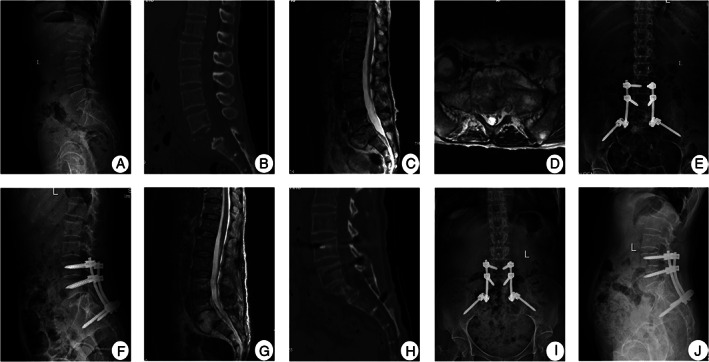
A 64‐year‐old female with L_5_/S_1_ TB underwent one‐stage posterior debridement, interbody fusion, and lumbopelvic fixation. (A–D) Preoperative images showing that the TB lesion was located at L_5_/S_1_ and the S_1_ vertebra was severely affected and collapsed, with prevertebral cold abscess formation. The lumbosacral angle was 24°. (E, F) Postoperative anterior and lateral radiographs of the lumbar spine demonstrating good position of posterior screw rod system, and lumbosacral angle was 27°. (G) Postoperative MRI showing most of abscess was eliminated. (H) CT showing bone fusion was obtained at 9 months. (I, J) X‐ray displaying good position of internal fixation, with a lumbosacral angle of 26° at the final follow‐up period of 72 months.
